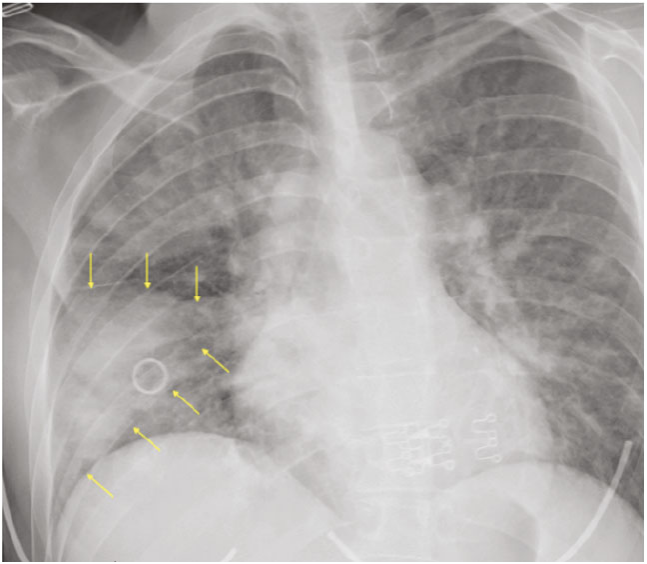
AP chest radiograph on initial presentation demonstrating a wedge like consolidation in the right-lower-lobe delineated by yellow arrows.
